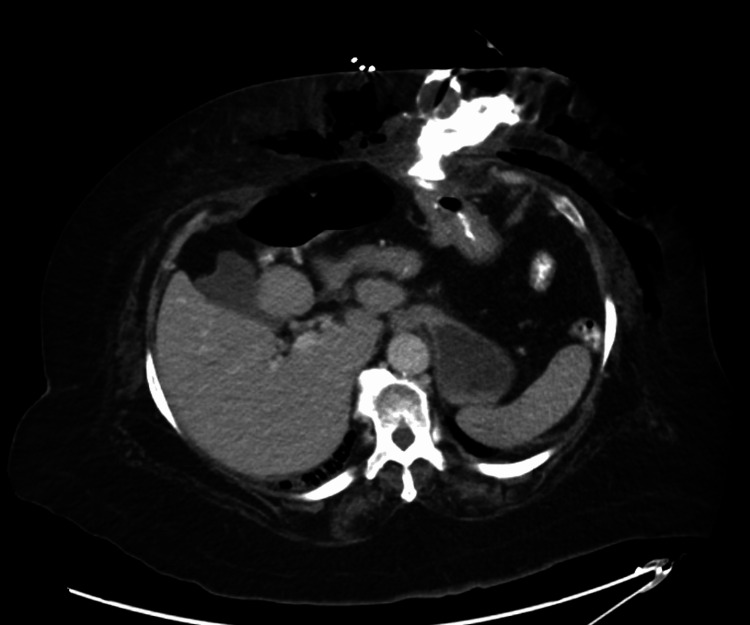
Computed tomography of the abdomen and pelvis with contrast that was taken at admission to the emergency department.
